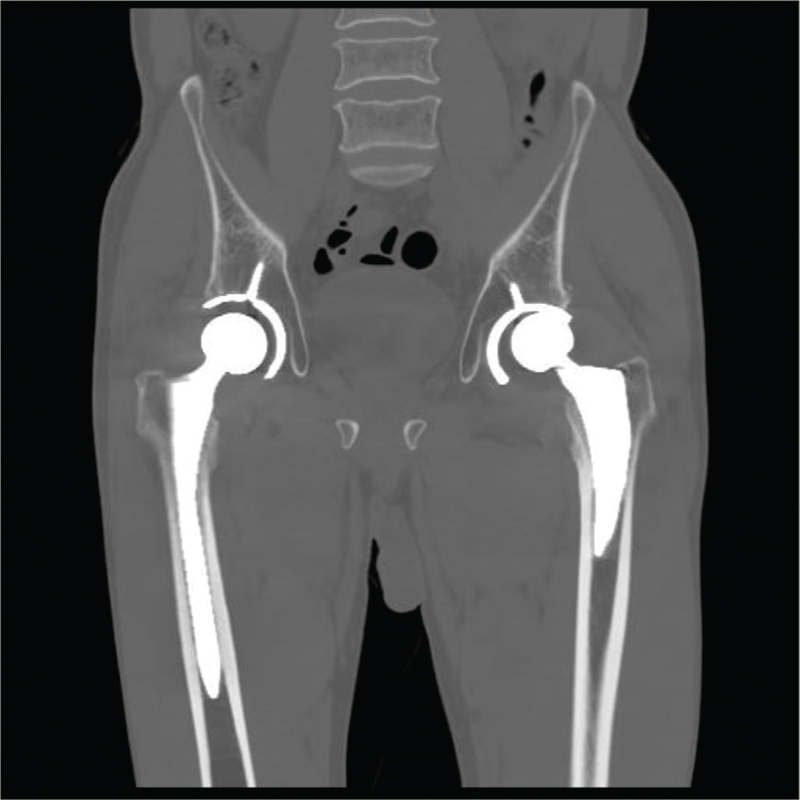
Metal artifact reduction computed tomography (MAR-CT) showing suspected polyethylene liner failure. MAR-CT = metal artifact reduction computed tomography.
